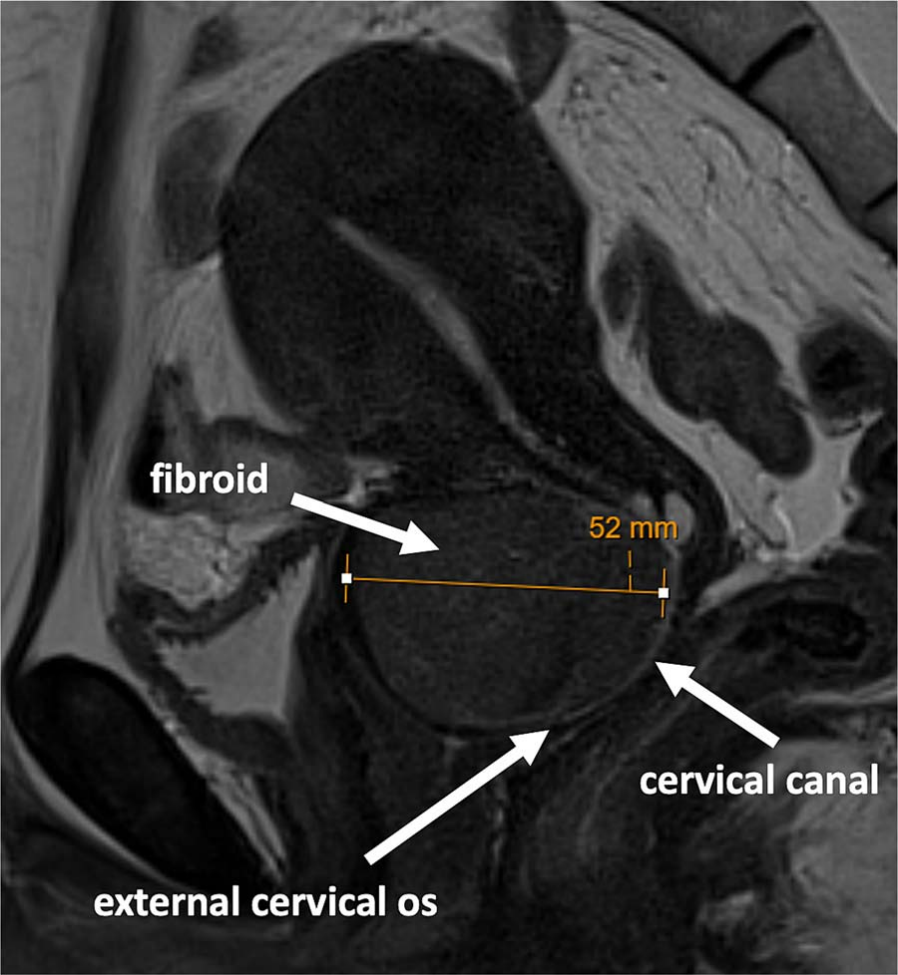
MRI scan showing large fibroid within the anterior cervical stroma with compression of the cervical canal.
